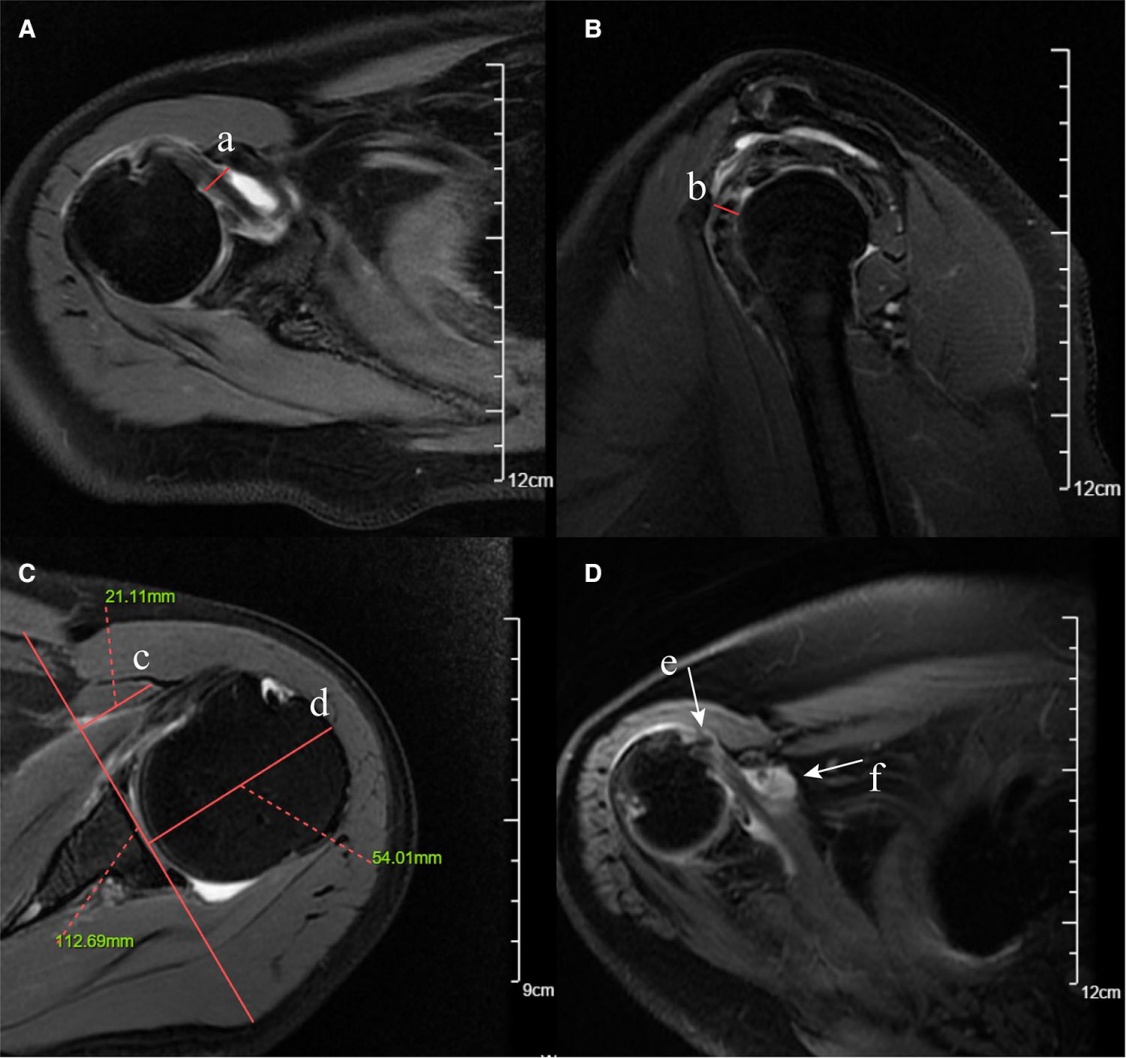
Fat-suppressed T2-weighted MRI images of coracohumeral distance (CHD), coracoid overlap (CO), coracohumeral index (CHI), LHB malposition, and subcoracoid effusion. (A) CHD measured on the axial plane (red solid line a). (B) CHD measured on the oblique sagittal plane (red solid line b). (C) CO (red solid line c) and CHI (red solid line c/ red solid line d, 21.11 mm/54.01 mm). (D) LHB malposition on the axial plane (white arrow e). (E) Subcoracoid effusion on the axial plane (white arrow f).
